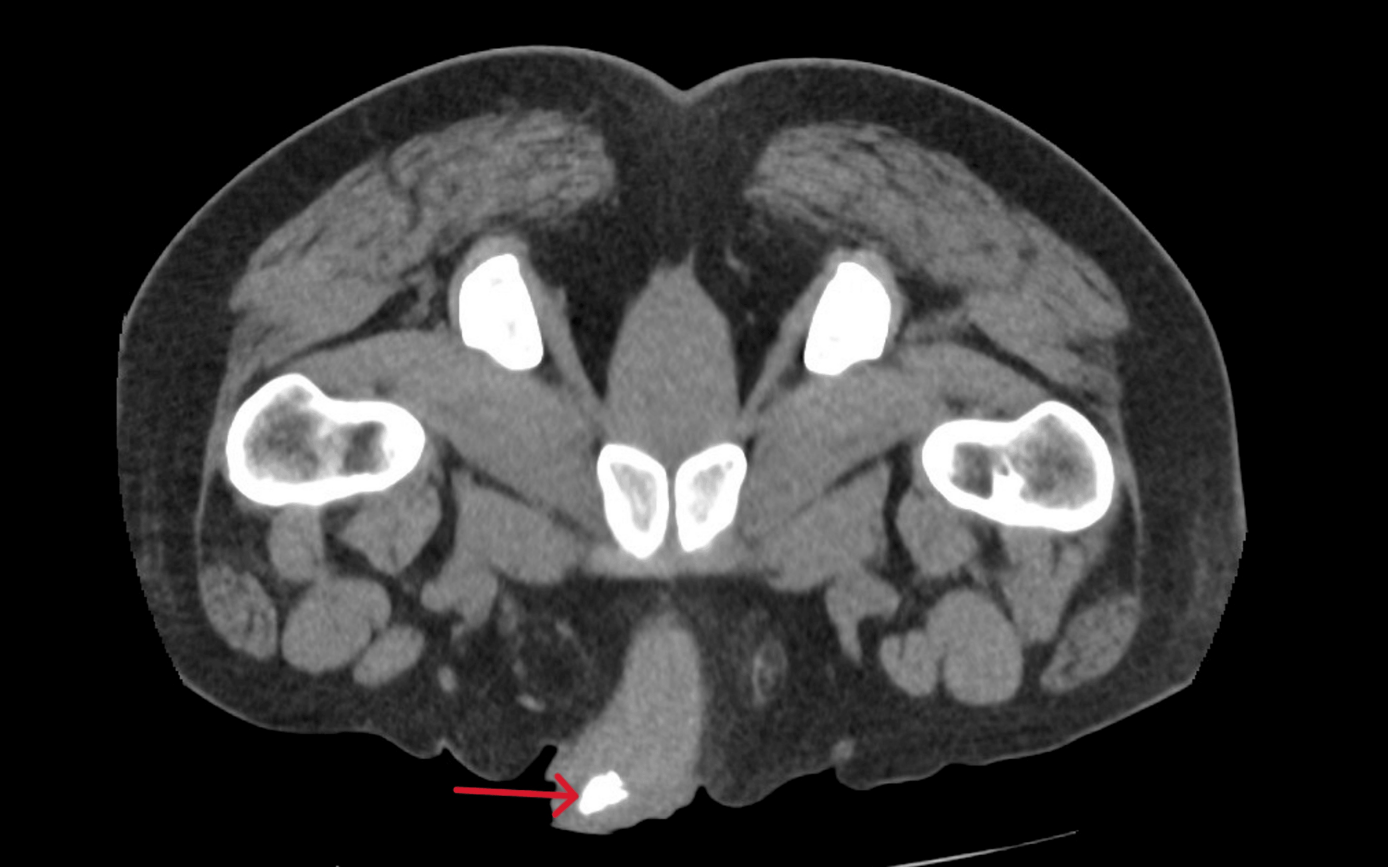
Computed tomography of the kidneys, ureters and bladder (CT KUB) showing a large calcification in the region of the urethra (red arrow).
